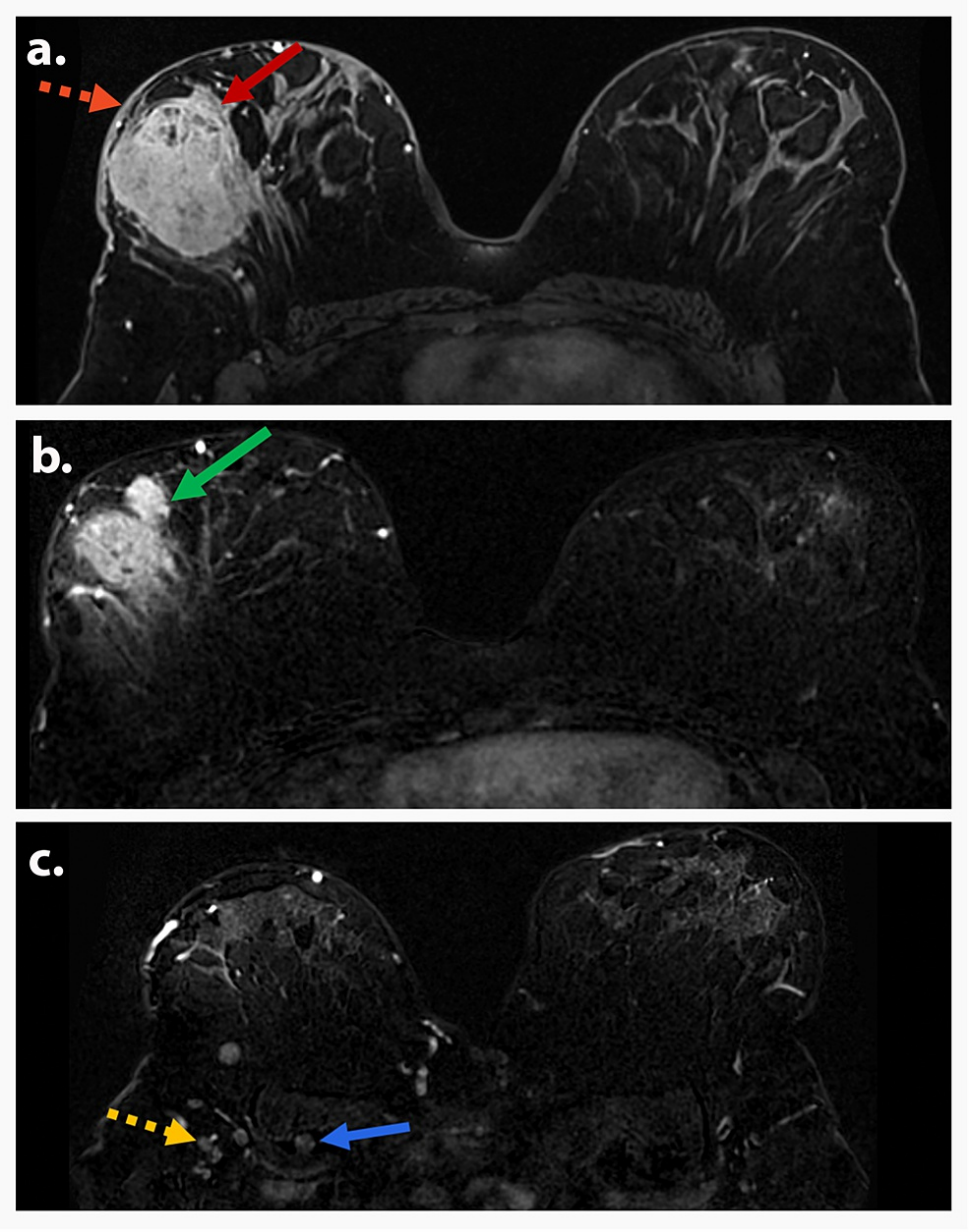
AJCC TNM stage IIIB (T4bN1M0) A 42-year-old female presented with a right breast mass, which on evaluation with a diagnostic mammogram, ultrasound, and subsequent biopsy was proven to be invasive ductal carcinoma. Axial postcontrast T1-weighted MR image (a) exhibits a 6.7 x 6 x 5.9 cm mass (red arrow) with heterogeneous enhancement in the lateral right breast with overlying thick and enhancing skin (dotted orange arrow), concerning for skin involvement (T4). Axial postcontrast T1-weighted subtraction MR image (b) shows a 2.2 x 1.9 x 1.7 cm enhancing mass (green arrow) at the anterior and inferior aspect of the index lesion at 9:00, 6 cm from the nipple. Axial postcontrast T1-weighted subtraction MR image (c) shows right axillary (dotted yellow arrow) and right sub-pectoral lymphadenopathy (blue arrow) (N1). Findings are consistent with AJCC TNM stage IIIB (T4bN1M0). AJCC: American Joint Committee on Cancer; TNM: tumor, node, and metastasis; MR: magnetic resonance; DCE: Dynamic contrast-enhanced.
